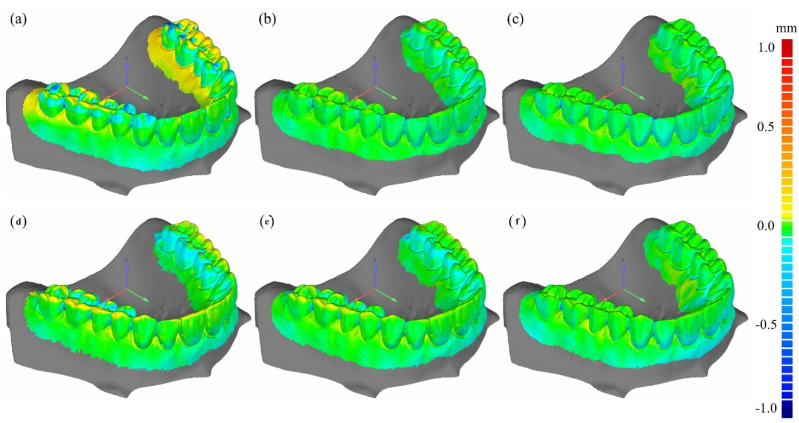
The deviation maps of Model 1 as compared to the optical STL (reference). (a–c) show deviation maps computed from the FDK images taken at the cone-beam angles of 10, 5, and 0 degrees, respectively, while (d–f) show deviation maps computed from the CW-FDK images taken at the cone-beam angles of 10, 5, and 0 degrees, respectively.
